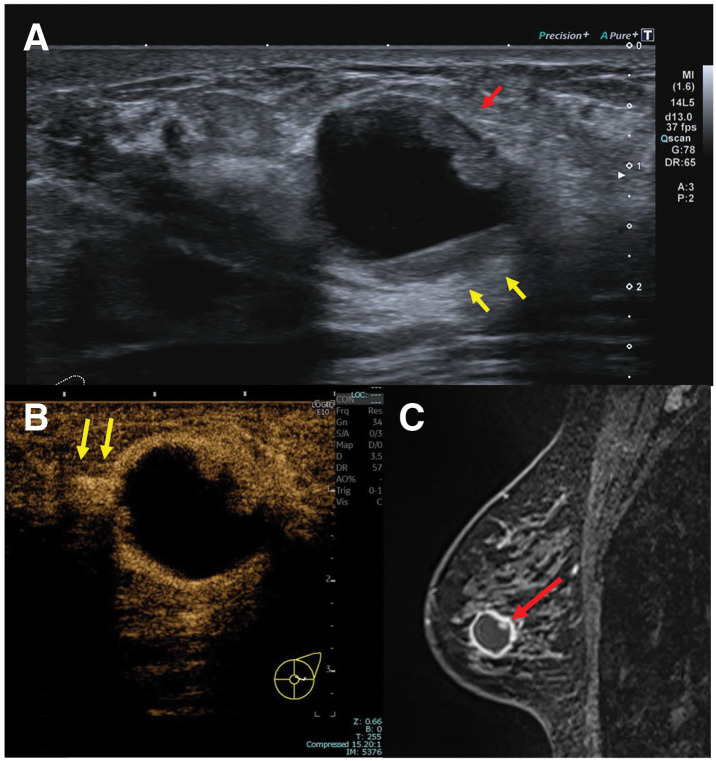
Imaging findings of Case 1. (A) B-mode US demonstrates a 20-mm intracystic mass with broad-based mural nodules (red arrow) and internal fluid–fluid levels (yellow arrows). (B) Contrast-enhanced US shows homogeneous enhancement of the cyst wall and mural nodules, with enhancement extending towards the nipple (yellow arrows) corresponding to the hypoechoic area on B-mode US. (C) MRI shows a cystic tumor with a homogeneously enhanced thickened wall and mural nodules (red arrow), consistent with the US findings. US, ultrasonography
